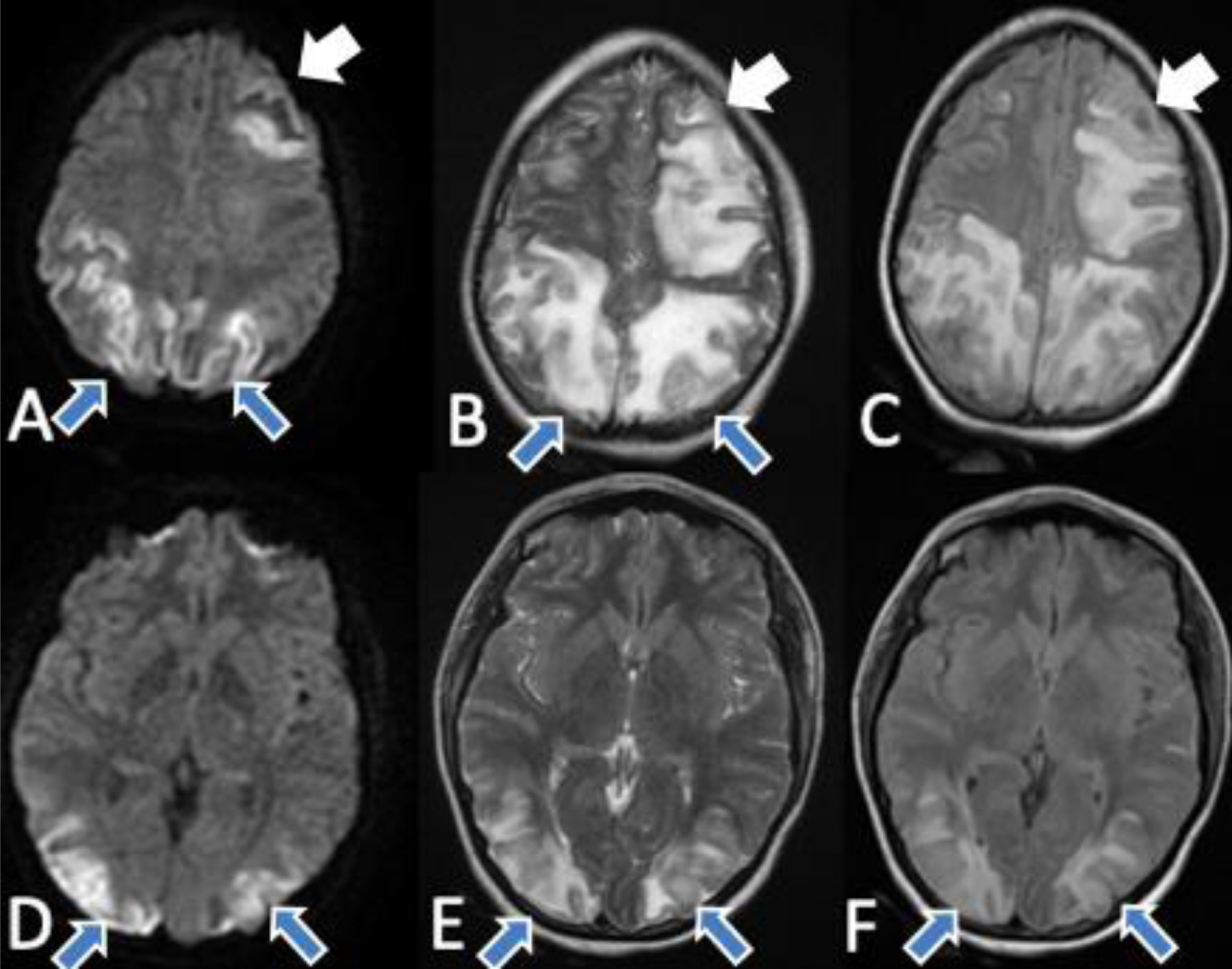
Brain MRI obtained on day 2 of admission, demonstrating extensive vasogenic edema with few areas of ischemic changes, in frontal (white arrows), parietal and occipital lobes (blue arrows), in diffusion sequences: (A-D), T2 sequences: (B-E), FLAIR sequences: (C-F) (Color version of figure is available online).
